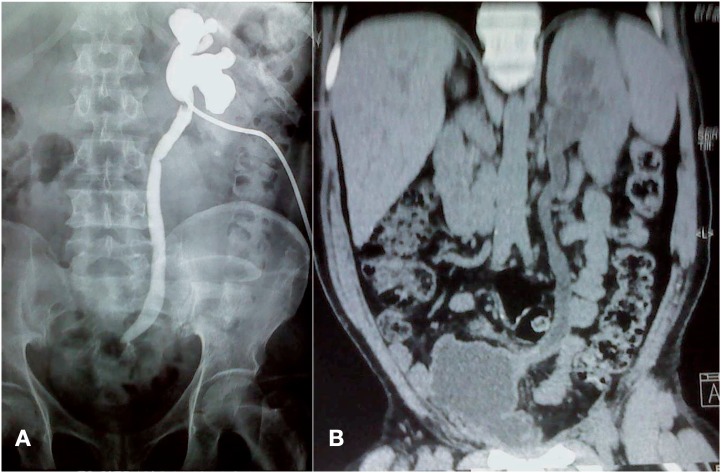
Uretero-ileal anastomotic stricture. (A) Antegrade urogram showing complete obstruction of the ureteroileal anastomotic stricture. (B) CT urography showing obstructed uretero-ileal anastomosis with dilatation of the renal unit.
